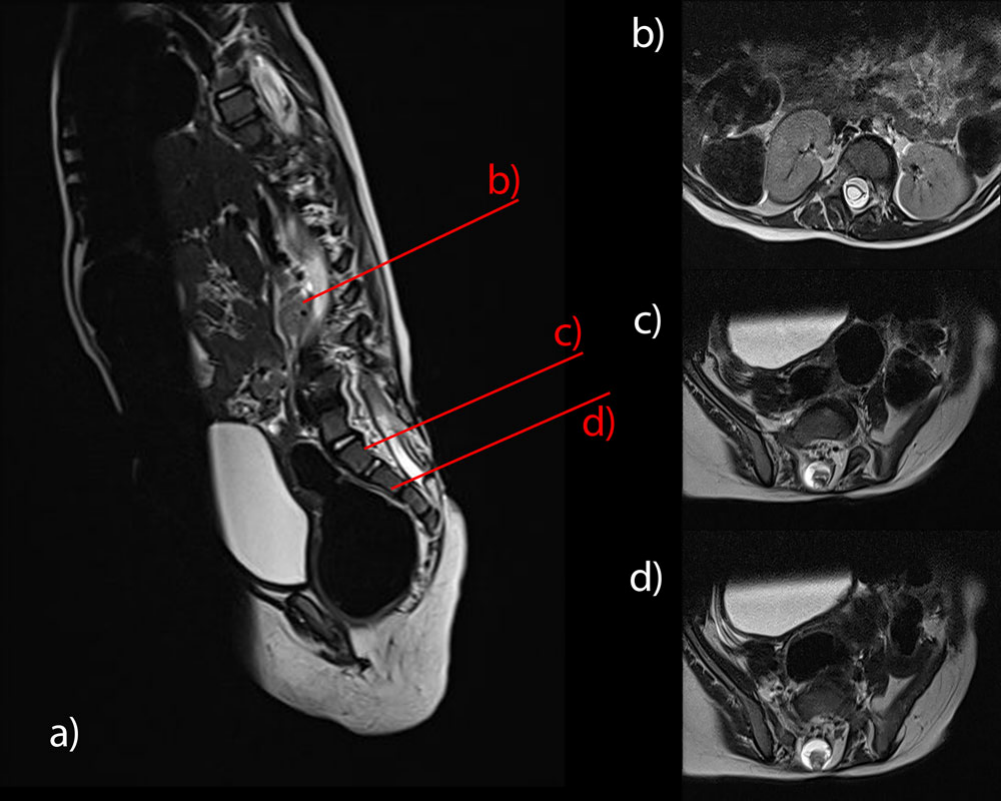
(a) Sagittal T2 MRI at age 1 year and corresponding axial T2 MRI slices, (b) at level of T11 with syringomyelia, (c) L5/S1 with dorsal lipoma and S1/2 with dermoid on the dorsal aspect of the placode with tethering.
